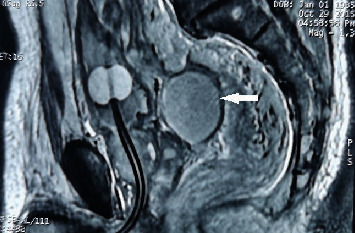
A mixed signal shadow (white arrow) can be seen as a quasielliptical on MRI T2WI, located between the rectum and bladder. The left high signal shadow (hollow in middle) is the water bag of the Foley catheter located in the bladder at the inner mouth of the urethra.
